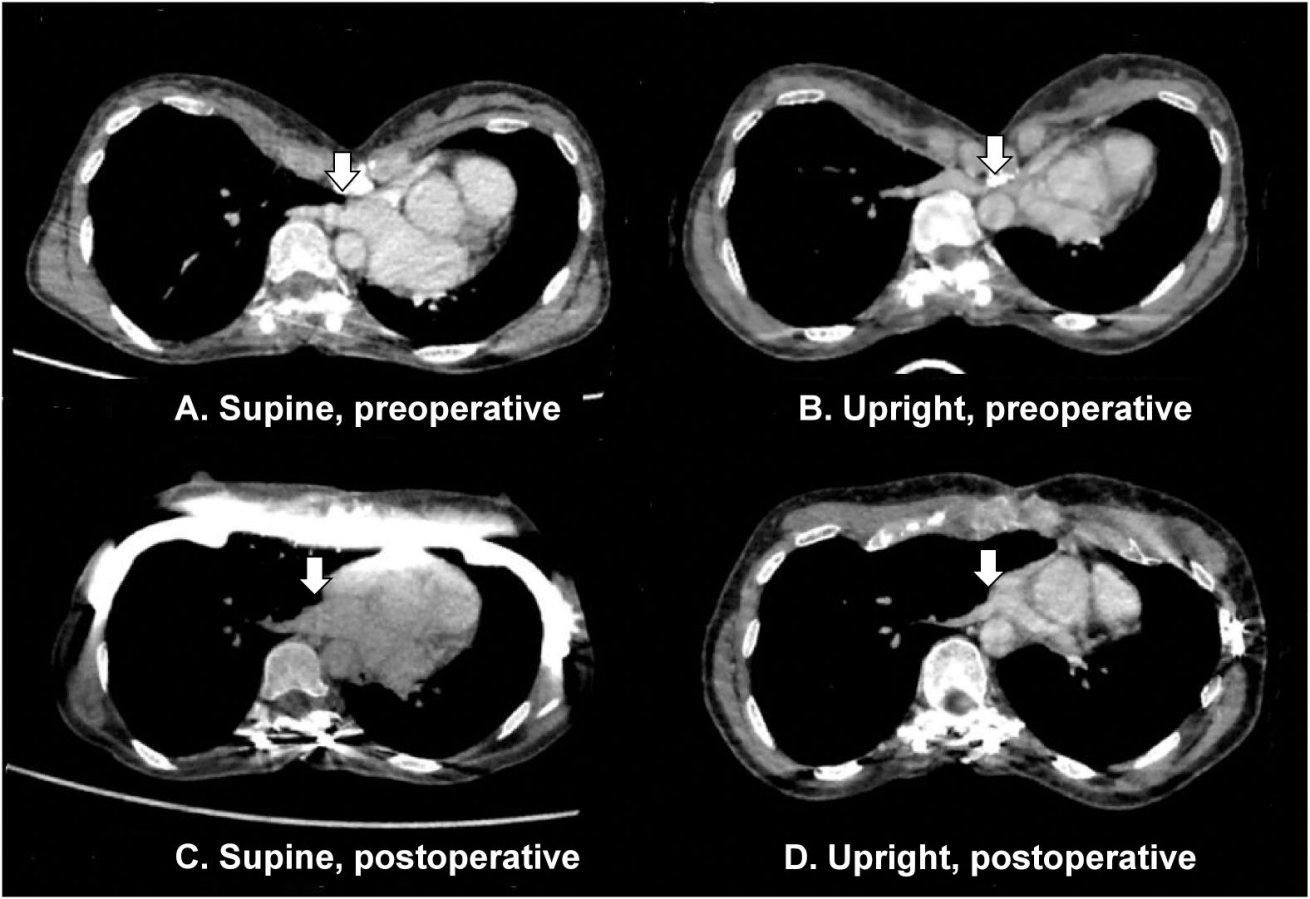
Horizontal computed tomography sections at the level of maximum chest depression from a 49-year-old patient with pectus excavatum presenting with dyspnoea, which worsened in the upright position. Images were captured during deep inspiration in the (A) supine position, preoperative; (B) upright position, preoperative; (C) supine position, postoperative; and (D) upright position, postoperative. The right inferior pulmonary vein (arrow) is significantly compressed in the upright position preoperatively (B).
